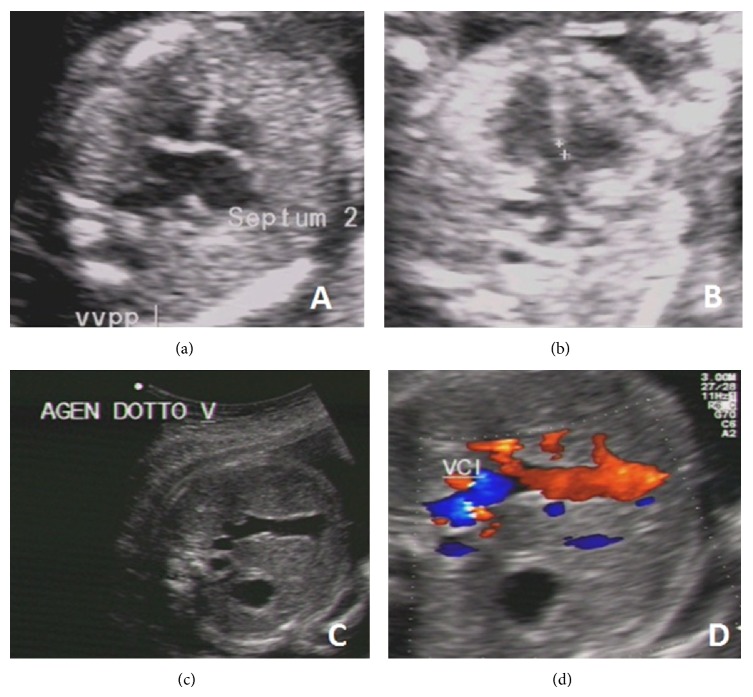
Prenatal ultrasonographic foetal heart anomalies detected at 19 ((a)* ostium primum* ASD; (b) perimembranous VSD) and 28 ((c)-(d) agenesis of the venous duct) weeks of pregnancy.
